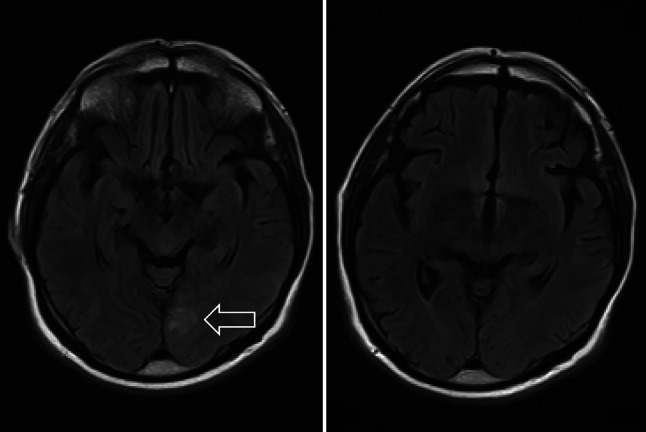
Amyloid-related imaging abnormality-edema (ARIA‑E; arrow) in a patient under administration of aducanumab, a monoclonal amyloid antibody which did not receive approval in Europe; shown is a Magnetic resonance imaging (MRI) with two axial sections, performed during the Phase 3 study Engage at the Medical University Graz. The left image shows ARIA - E in the left occipital lobe, the right image shows complete remission after discontinuation of the therapy within 1 month
